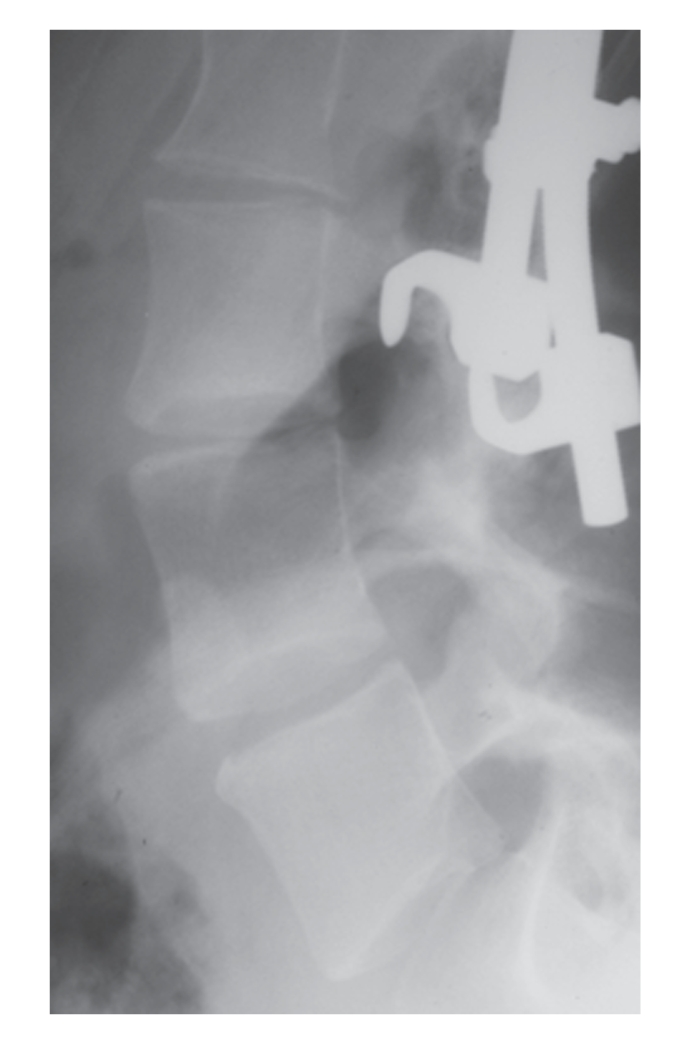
Radiography of the lumbal spine demonstrating osteosynthetic material until the border of L3.
